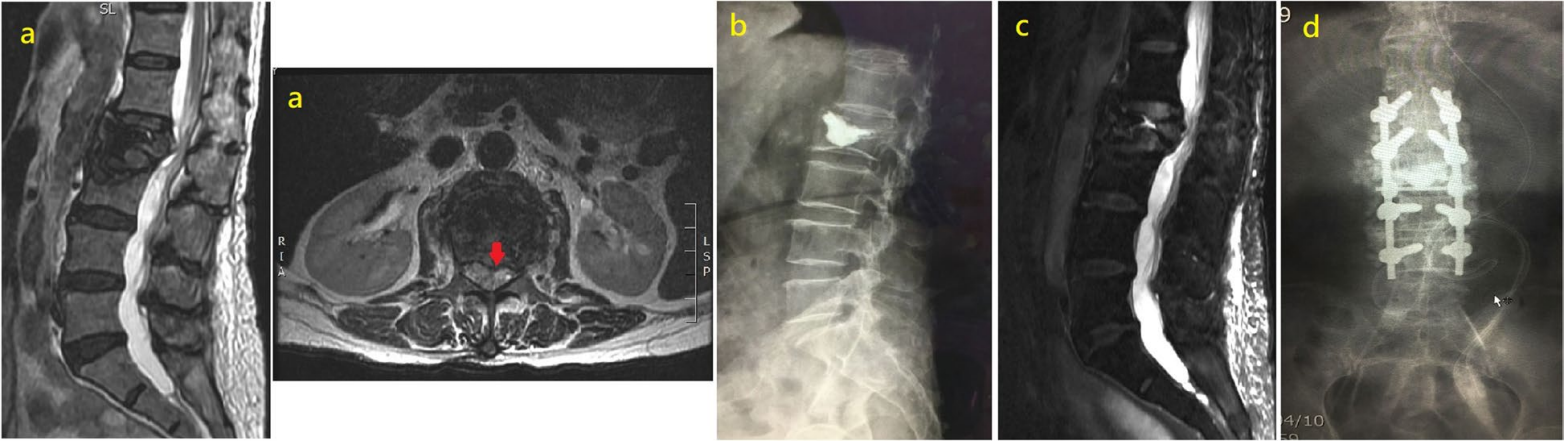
MRI of L2 compression fracture with posterior vertebral cortex disruption before PKP (red arrow) (a). We performed L2 PKP with 4.0 c.c. bone cement (b). MRI showing spinal canal compromised by a retropulsed bone fragment after balloon kyphoplasty (c). L2 level posterior decompression (PD) and T12-L4 instrumentation (PI) with pedicle screws (d)
